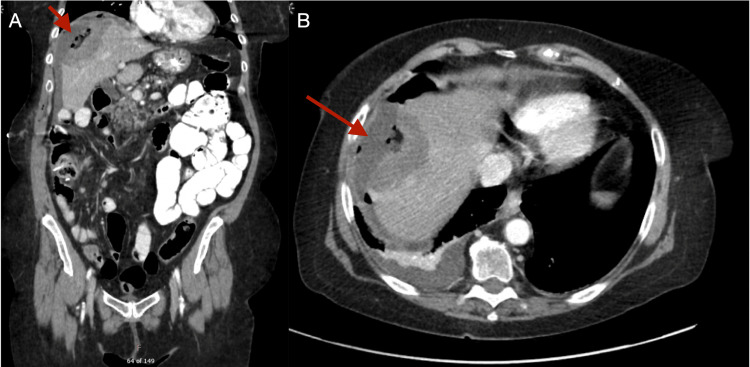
CT of the abdomen and pelvis with the portal venous phase of contrast and oral contrast enhancement performed three days post RFA showing a 6.2 × 4.1 × 7 cm area of low attenuation with associated fluid and pneumatosis. (A) Coronal view. (B) Axial view. CT: computed tomography; RFA: radiofrequency ablation
